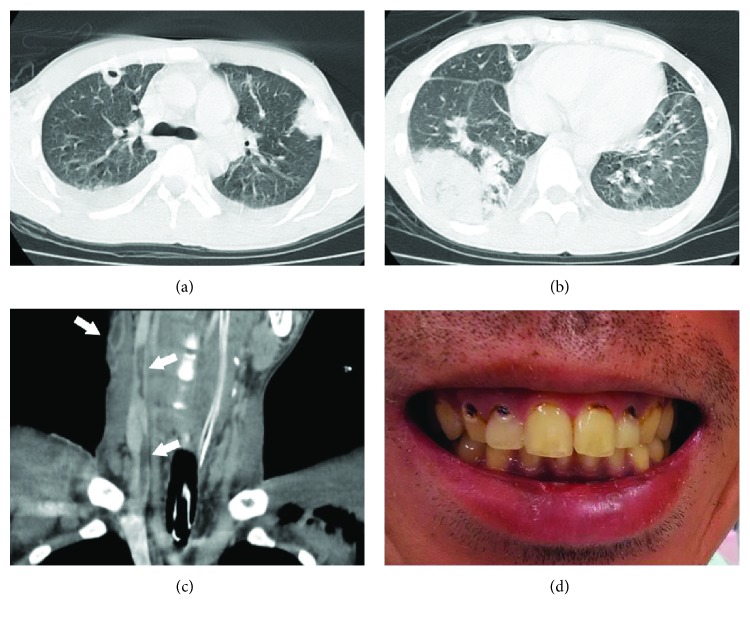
(a, b) Computed tomography undertaken upon hospital admission (pulmonary window setting). Multiple bilateral peripheral pulmonary masses with cavitation and pleural effusion can be observed. (c) Contrast-enhanced computed tomography. Thrombosis of the right internal and external jugular vein can be observed (arrows). (d) Intraoral examination. Periodontal disease and multiple caries are revealed in the oral cavity.
